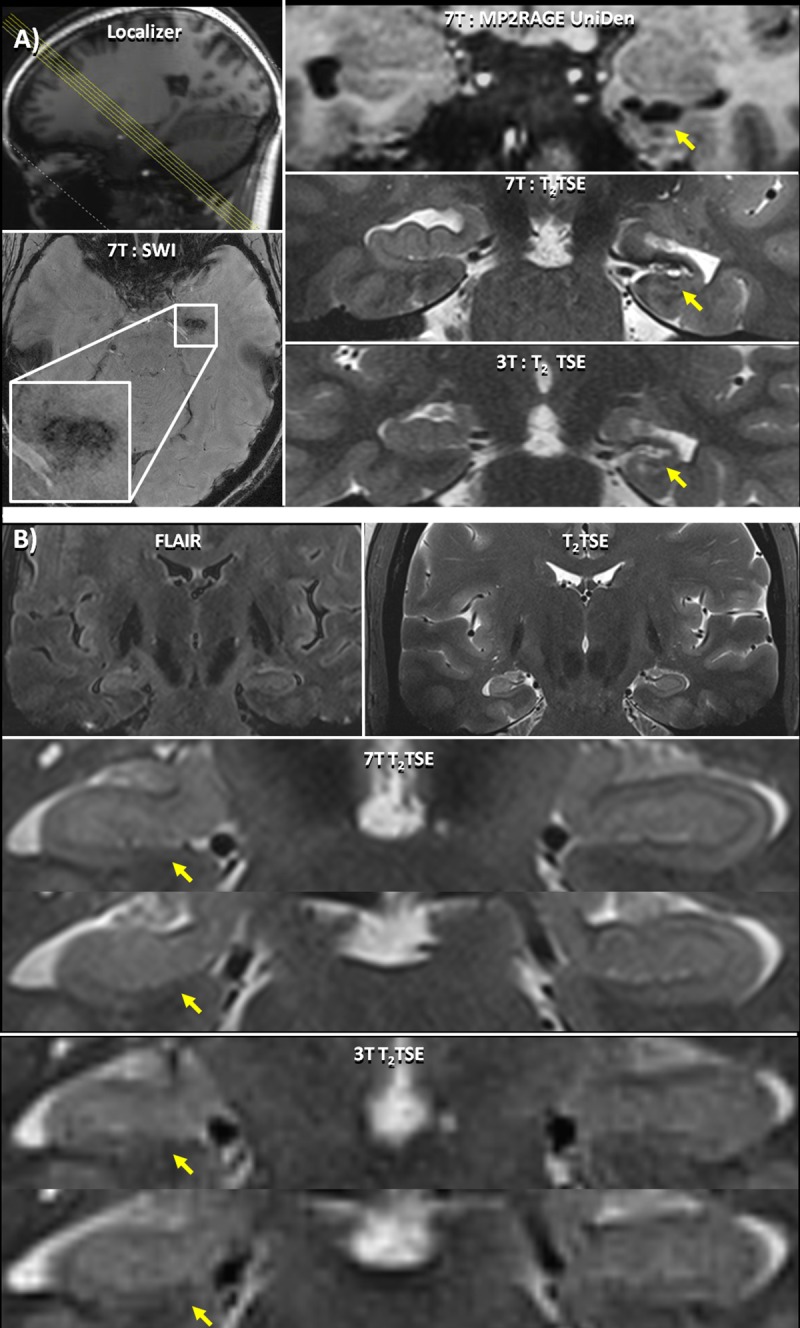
Hippocampal Asymmetry. (A) Patient 19 –clockwise from top left: A low resolution localizer indicating the coronal-oblique slice thorough the hippocampus shown; 7T: MP2RAGE UniDen reconstruction visualizing the cavity; 7T:T_2_ TSE image showing a coronal oblique slice through the hippocampus and a visualization of the parenchymal cavernoma; 3T: T_2_ TSE scan, acquired previously, showing the location of the lesion. On the 3T image, the lesion was less conspicuous and therefore went undiagnosed despite being identified in a retrospective examination of the image; SWI axial slice where the cavernoma can be clearly identified. (B) Patient 24—from top left: A 7T FLAIR image showing relatively equivalent signal intensity in both hippocampi; 7T:T_2_ TSE image showing full coronal-oblique slice and right hippocampal sclerosis, and 3T T_2_ images showing the hippocampus.7T:T_2_ TSE slice series showing a coronal oblique slice through the hippocampus showing right hippocampal sclerosis with decreased digitation and lamination without accompanying signal change in the hippocampus on the FLAIR image. The 3T T_2_ images for this subject do not show this architectural change in the hippocampus.
